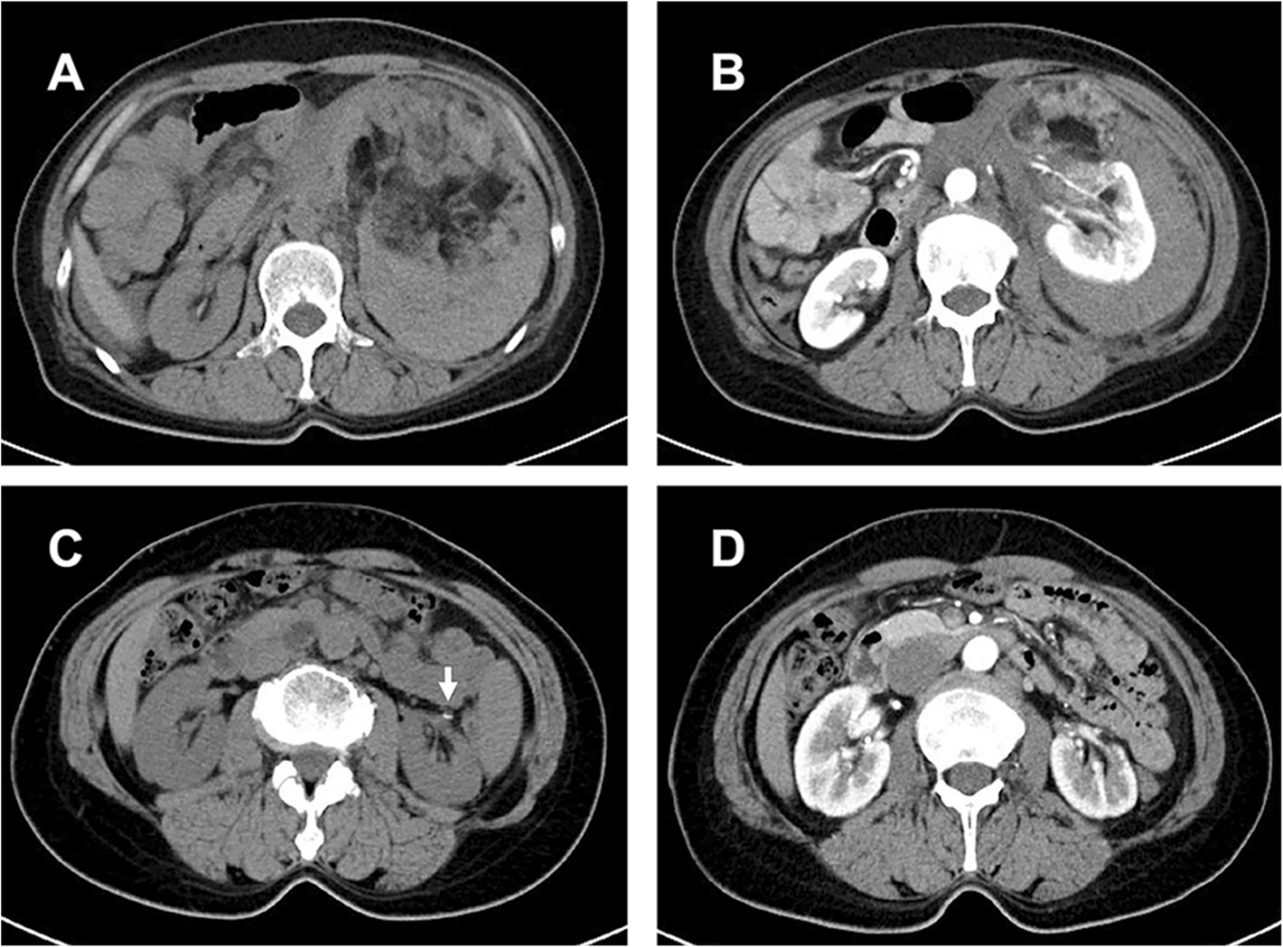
A ruptured left renal AML. The preoperative CT scans (a and b) show the abnormal morphology of the left kidney with a mixture of adipose tissue, ruptured cortex and retroperitoneal hematoma. The postoperative CT scans (c and d) (1 year after surgery) show left renal cortex defect and the hem-o-loc clip (white arrow). The ruptured renal AML was resected completely, and no recurrence was found
